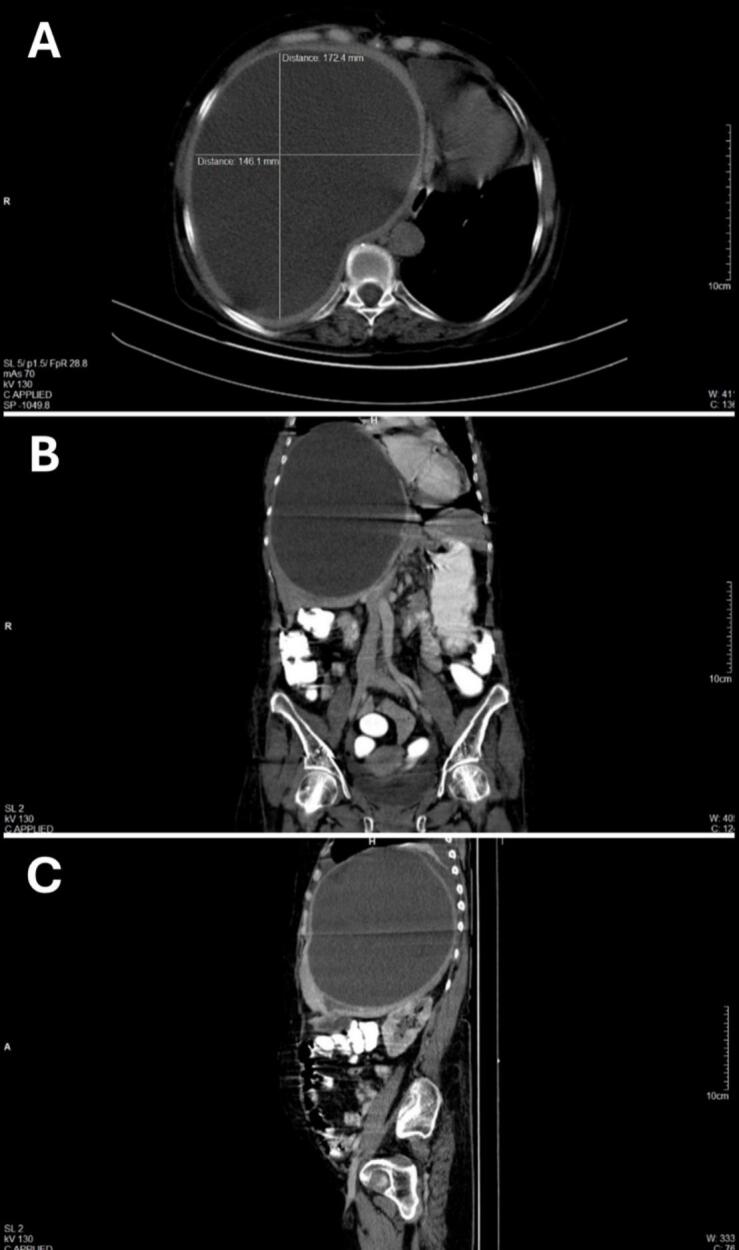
Triple phase CE-CT scan of the abdomen- (A) Axial, (B) coronal and (C) sagittal views showing a hypoechoic cystic lesion involving the right lobe of the liver.
